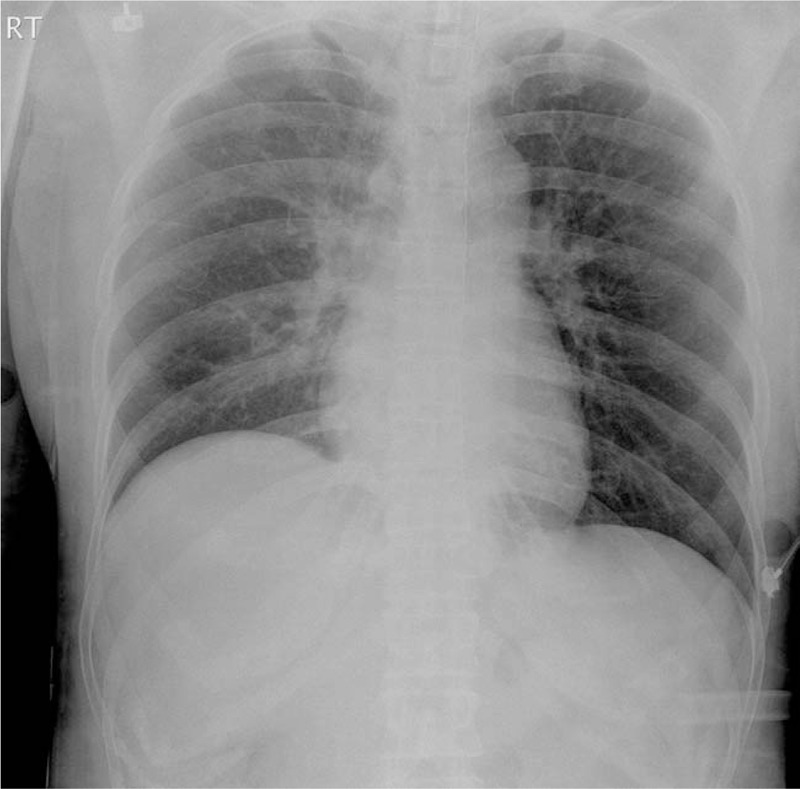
Chest radiography after removing the central venous catheter.
